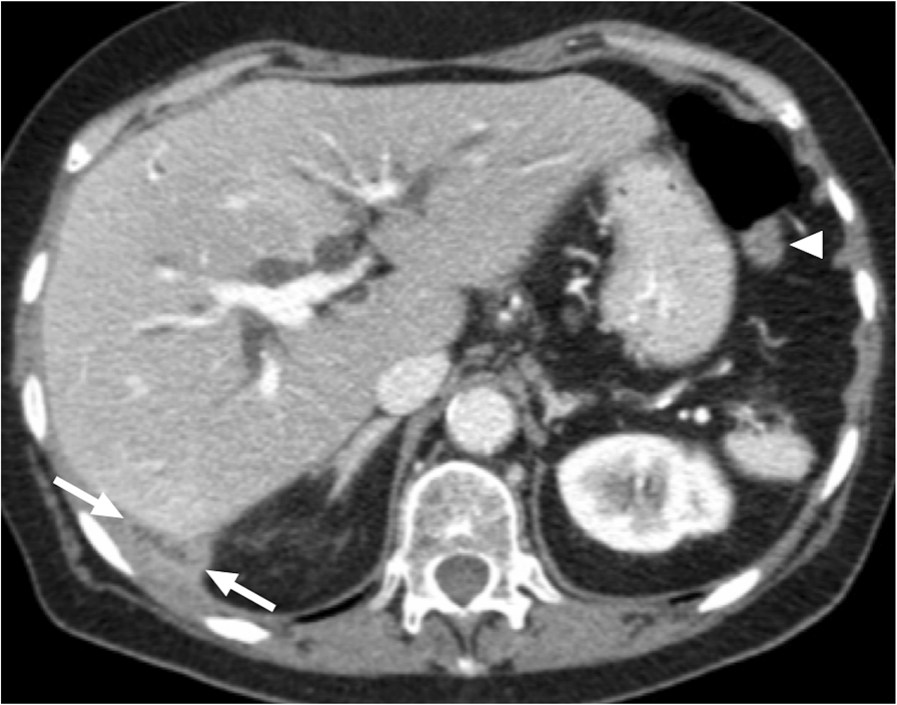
A 65-year-old male with a history of colon cancer in remission for 7 years underwent a follow up CT. He was completely asymptomatic at the time of the CT scan. He also has a remote history of laparoscopic cholecystectomy 5 years before. Axial plane postcontrast CT image demonstrates a complex looking cystic mass in the liver capsule (arrows) suggestive for a tumor implant. Also note is made of a small solid nodule in the left upper quadrant (arrowhead). Surgical excision confirmed malignant implant in the left upper quadrant and an inflammatory pseudomass in the liver capsule due to a dropped gallstone.
